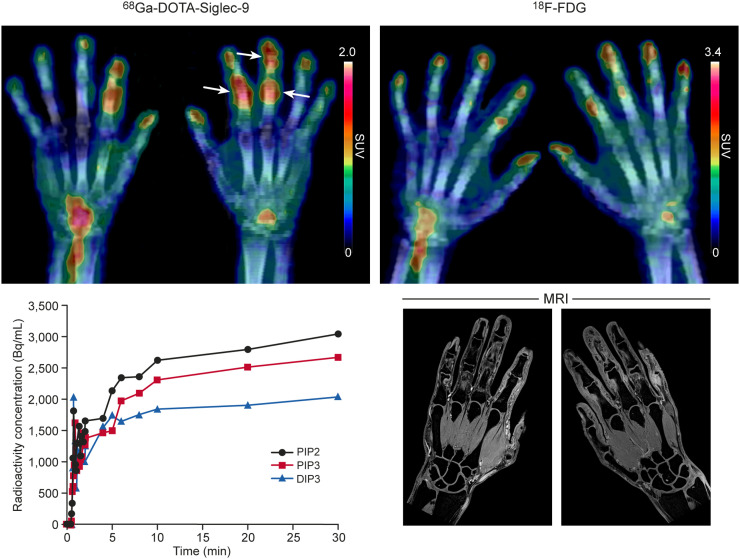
^68^Ga-DOTA-Siglec-9 and ^18^F-FDG PET/CT and MR images of hands of 49-y-old patient with early RA. Arrows denote proximal interphalangeal joints PIP2 and PIP3 and distal interphalangeal joint DIP3, whose time–activity curves are at bottom left. Respective SUVs were 1.05, 0.92, and 0.70 with ^68^Ga-DOTA-Siglec-9 and 1.46, 1.22. and 1.08 with ^18^F-FDG.
